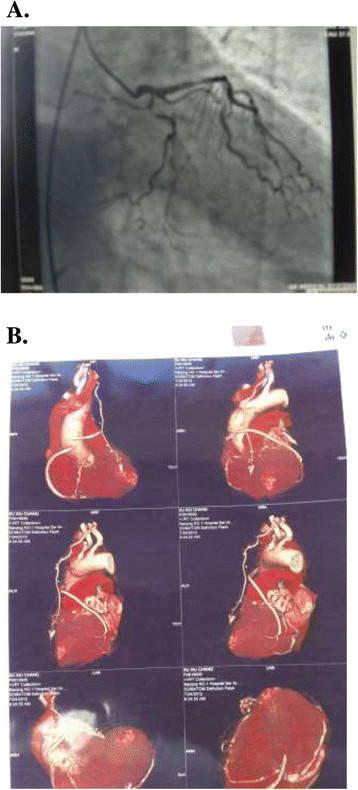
Preoperative and Postoperative follow-up angiogram study. (A) Preoperative angiogram demonstrates a diffusely diseased left anterior descending coronary artery (LAD). (B) Postoperative follow-up computed tomography (CT) angiogram showing patency of the left internal mammary artery to the LAD grafts.
